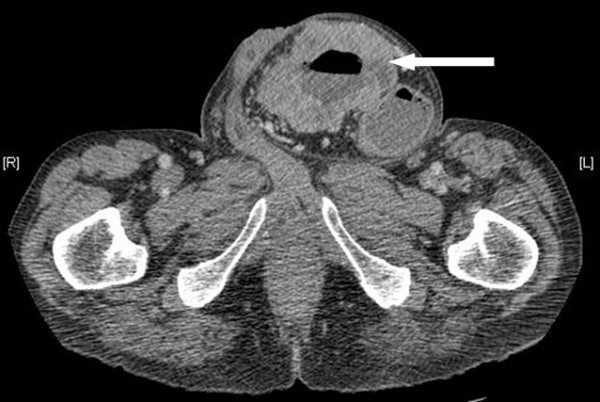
Imaging of the abdomen (cross sectional). CT scan showing tumour of the transverse colon (white arrow) presenting within a left inguinoscrotal hernia.
